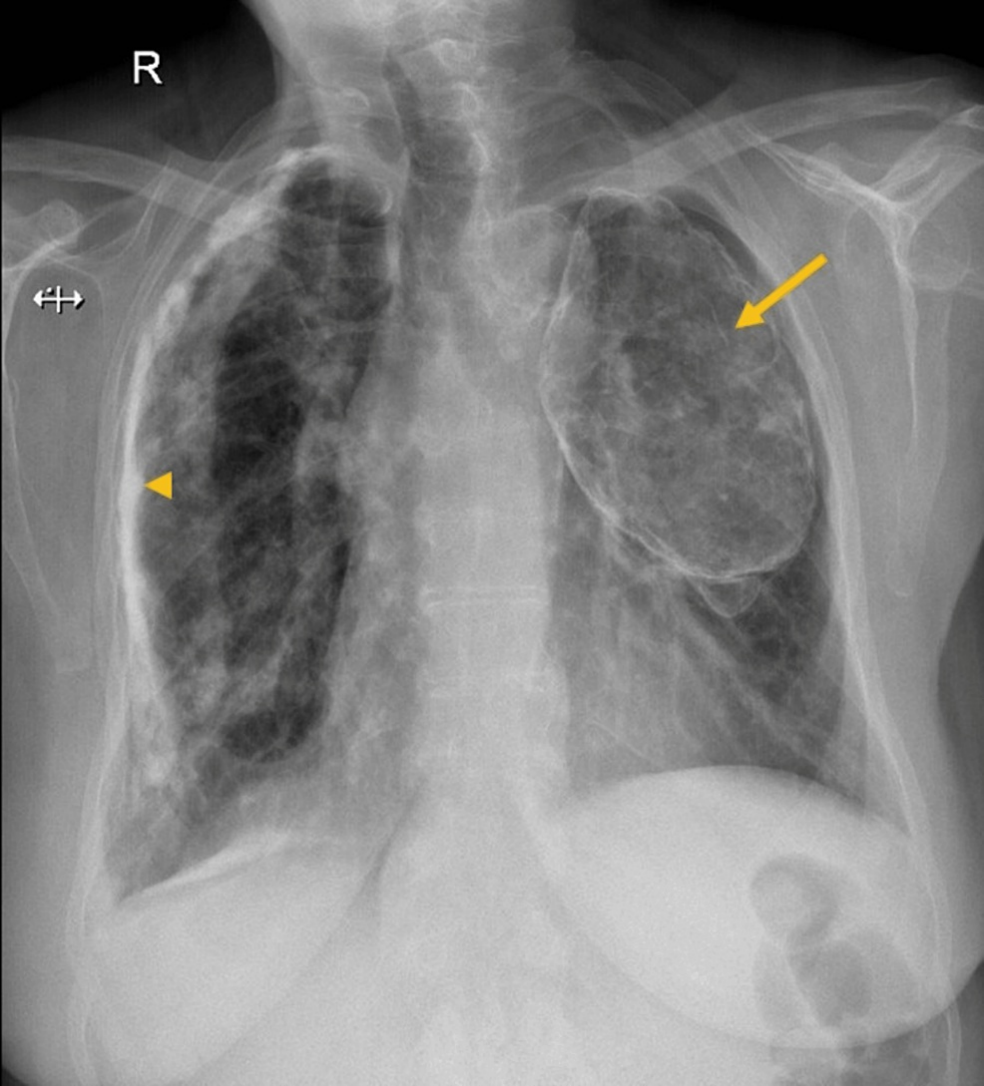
Posteroanterior chest radiograph showing a calcified oleothorax (arrow) and calcified pleural plaques from tuberculosis pleurisy (arrowhead)
